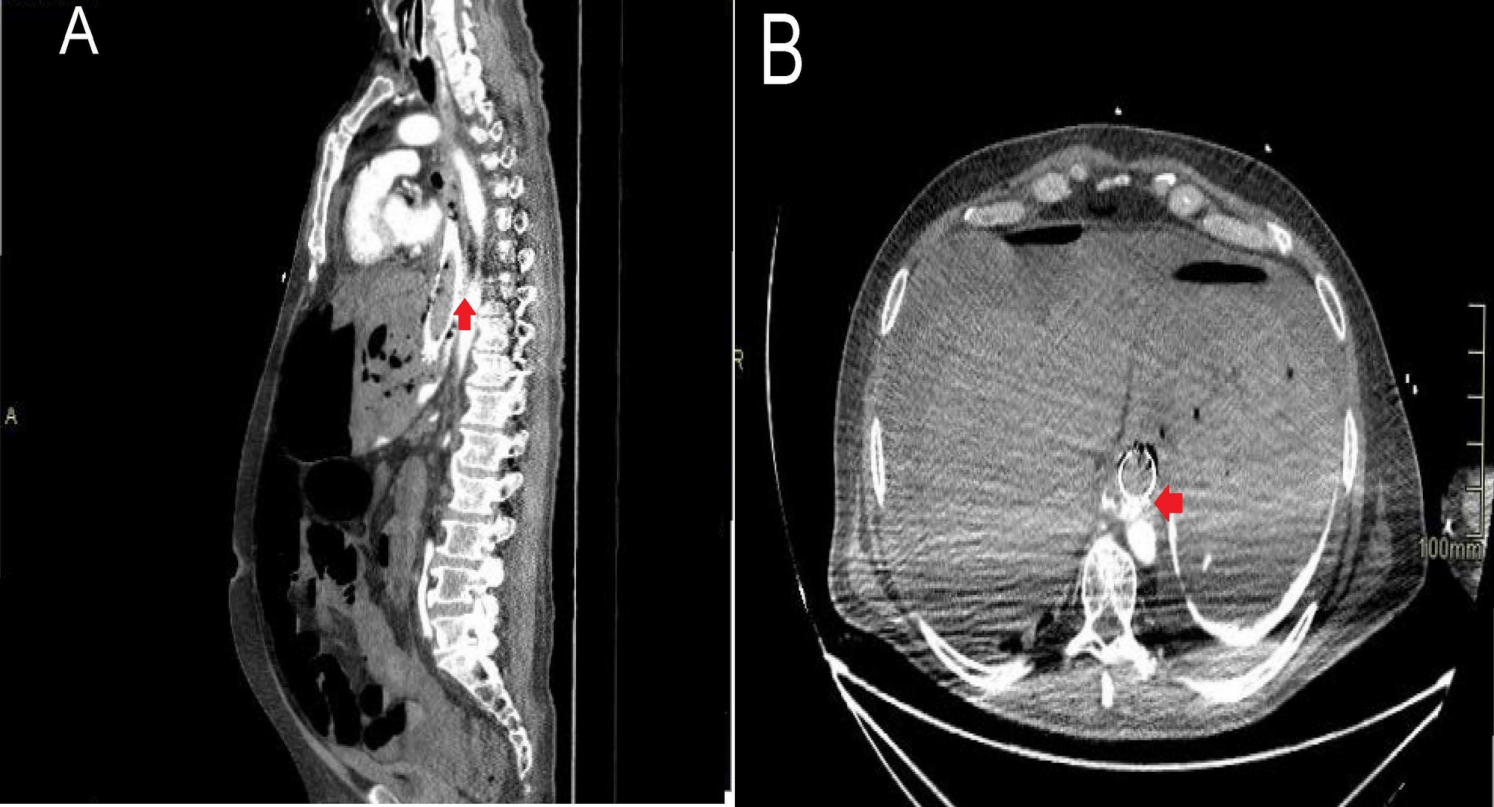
CT angiogram (A) Sagittal CT angiogram showing the leakage of contrast to the esophagus; (B) Cross-sectional CT angiogram showing contrast leak to the esophagus
